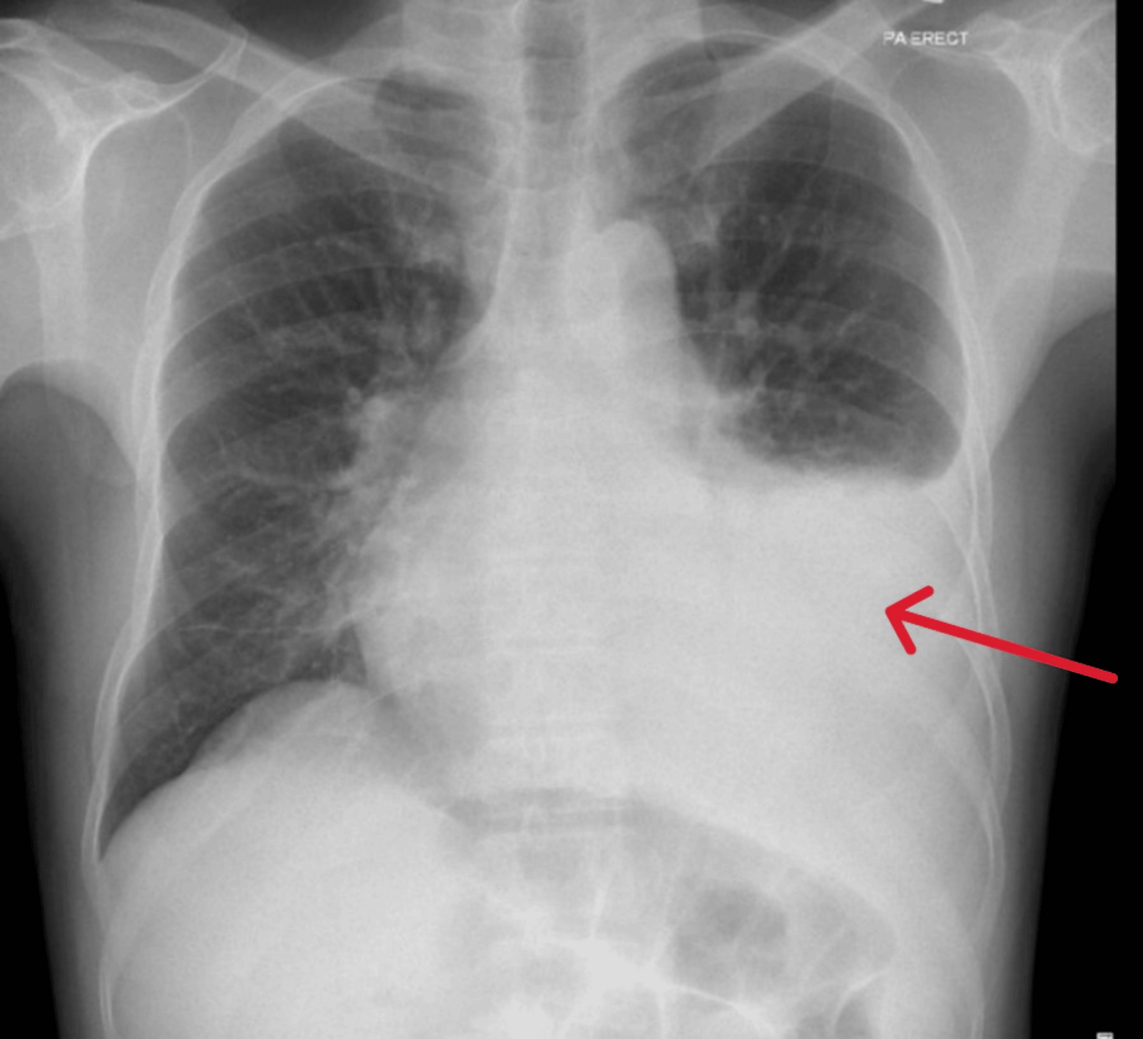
Chest X-ray (posteroanterior view) showing left-sided pleural effusion (red arrow).
